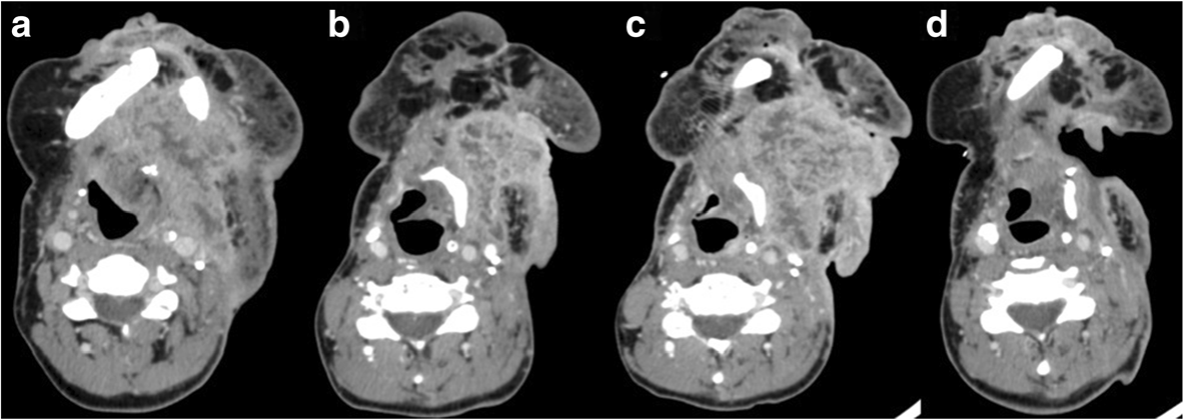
Change in largest dimensions of neck mass on CT scans over treatment period. a Prior to pembrolizumab. 8.8 × 5.9 cm. b Best response to pembrolizumab. 6 × 4 cm. c Progression on pembrolizumab. 7.1 × 7.2 cm. d Post radiation 5.9 × 3.4 cm
